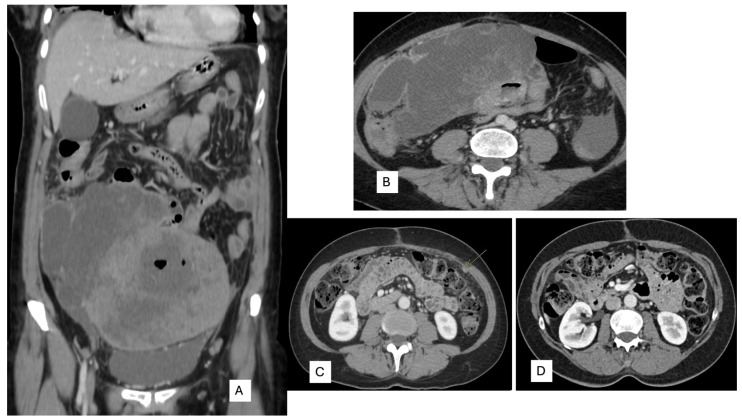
(A) Coronal and (B) axial. Computerized tomography displays a large intra-abdominal and pelvic tumor with necrosis attached to the small bowel. (C) Peritoneal carcinomatosis (arrow) in CT scan of follow-up. (D) CT scan after 3 months with Entrectinic treatment, showing a complete response.
